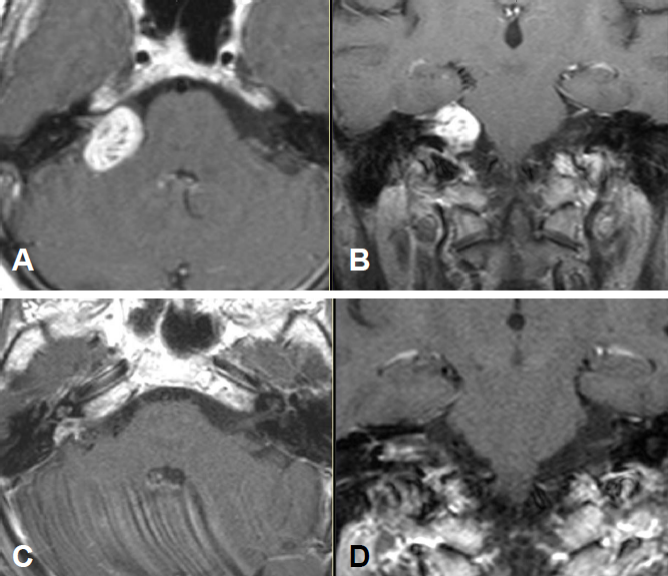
Preoperative MRI, T1-w + CM; A transversal; B coronal. The tumor reaches the fundus of the internal auditory meatus and the brainstem. MRI 4 months after surgery, T1-w + CM; C transversal; D coronal.
